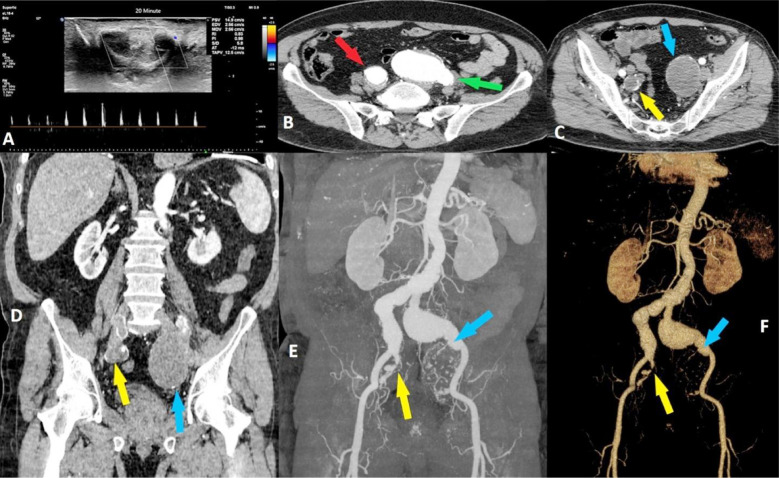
The peak systolic velocity was still around 10-15 cm/sec in post-injection Doppler images obtained at the 20th minute. The cavernous artery was measured 0.4 mm in diameter before injection and no enlargement occurred after the injection. No tumescence and partial rigidity were observed visually. Fig.1(B): Axial computerized tomography section indicating aneurysmatic right and left common iliac arteries with contrast agent included. Fig.1(C): Axial computerized tomography section pointing aneurysmatic right and left internal iliac arteries without contrast agent verifying the complete and almost complete blood flow blockage in the left and right internal iliac arteries respectively. Fig.1(D): Imaging of the explanation in C on the coronal section. Fig.1(E): Aneurysm formations on coronal maximum intensity projection images with almost completely obstructed right internal iliac artery and bo flow in the right internal artery. Fig.1(F): Imaging of the explanation in E in three-dimensional mode. Red arrow: right common iliac artery, green arrow: left common iliac artery, yellow arrow: right internal iliac artery, blue arrow: left internal iliac artery.
